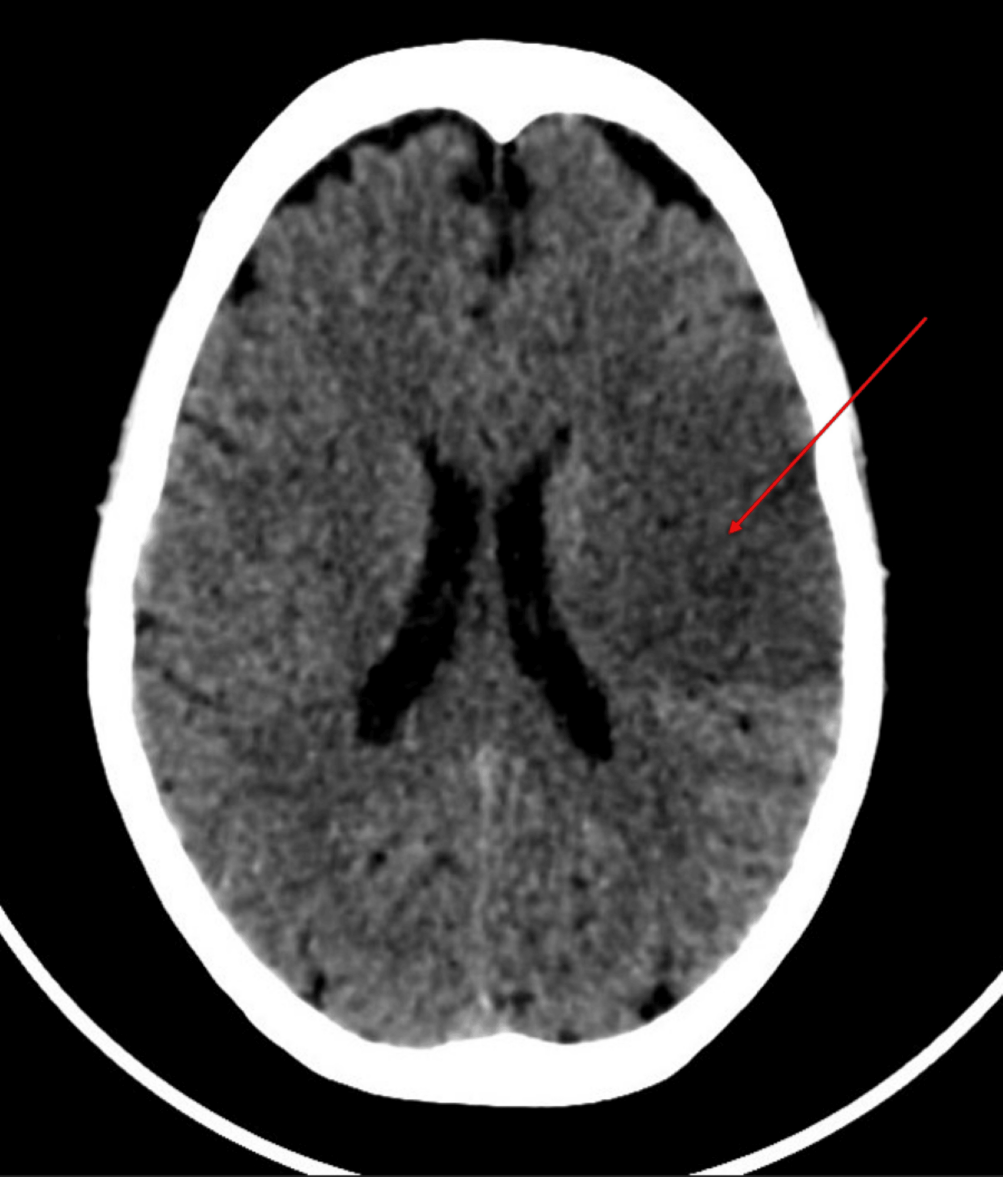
CT scan of the brain showing acute non-hemorrhagic infarct in the left fronto-parietal region (red arrow)
